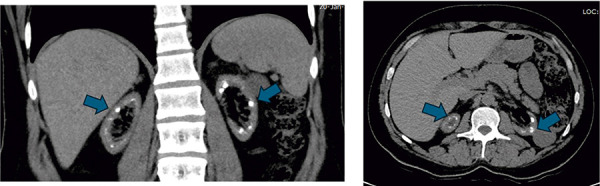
Non-contrast CT abdomen suggestive of bilateral medullary nephrocalcinosis (blue arrows). A: Sagittal section. B: Coronal section. CT abdomen and pelvis: medullary nephrocalcinosis (blue arrows).
